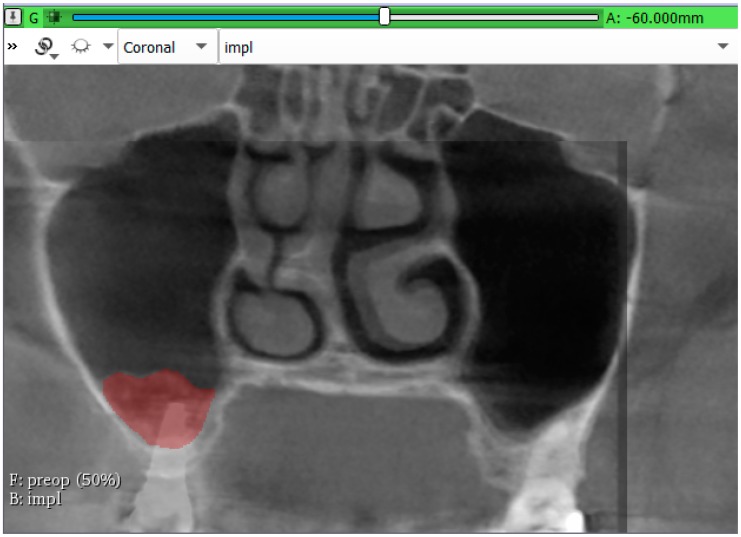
Volume measurement was carried out in the coronal section of fused images by changing the opacity of the layers of the pre- and postoperative CBCT, and—in this specific image—preoperative and 3-year control CBCT data of the same patient.
